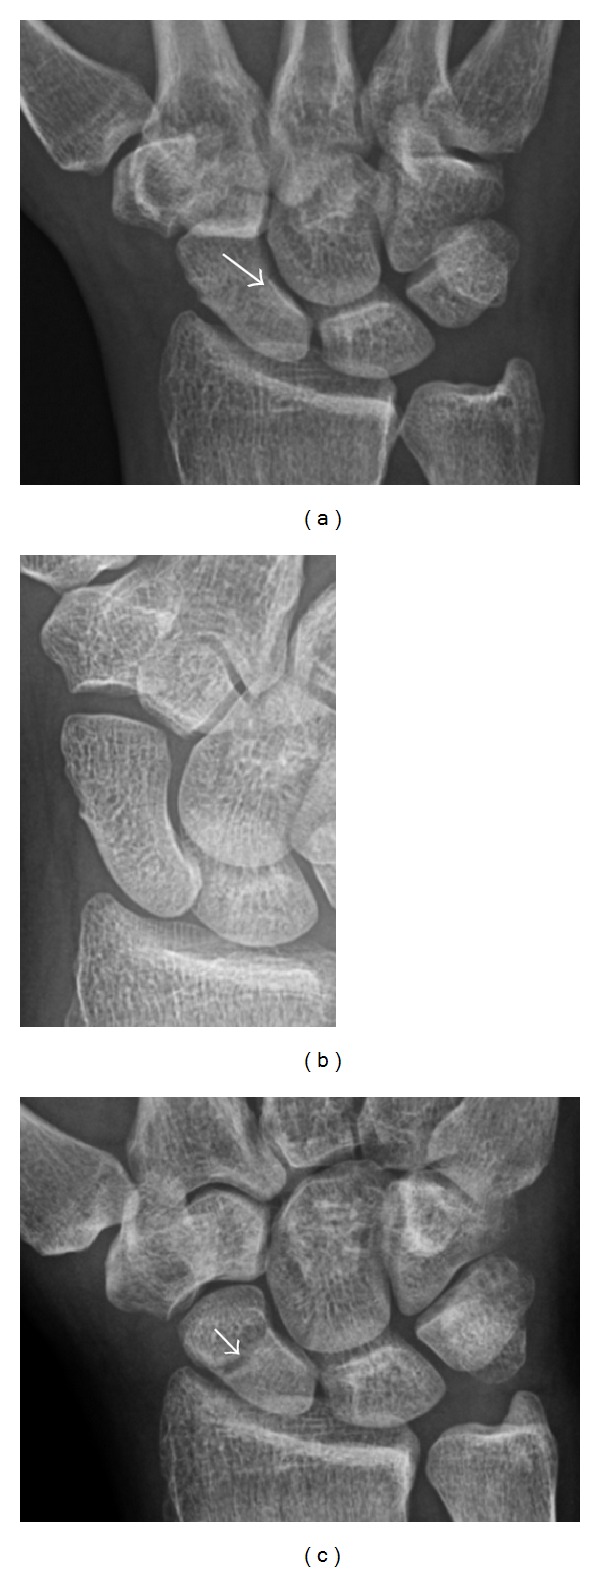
A 26-year-old man presenting with wrist pain after being assaulted. (a) Initial anteroposterior radiograph shows a subtle linear lucency within the scaphoid extending to the scaphocapitate articular surface that was overlooked (arrow). (b) Initial “scaphoid” view was negative. (c) Followup anteroposterior radiographs, 12 days later, shows obvious scaphoid fracture (arrows).
